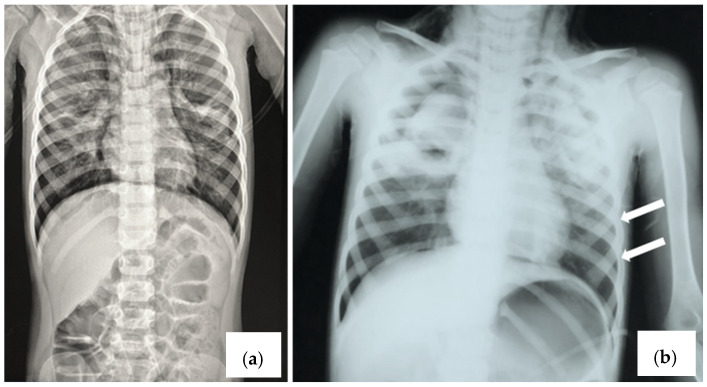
Chest X-ray (anterior–posterior) showing (a) diffuse interstitial infiltrate; (b) left pneumothorax indicated with arrows.
